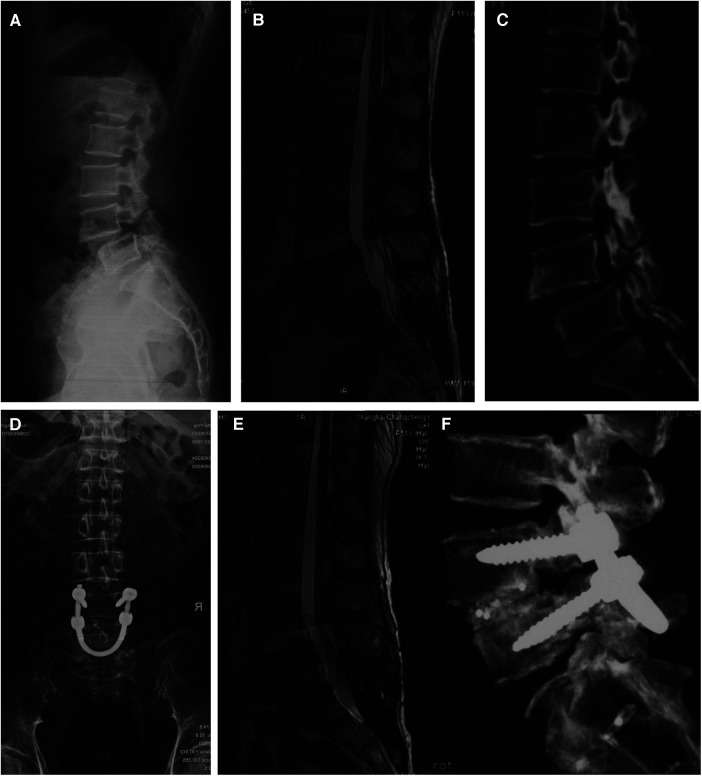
Preoperative and postoperative images obtained in a 51-year-old female diagnosed with double-level lumbar spondylolysis. (A–C) Spondylolysis at L4 and L5, and spondylolisthesis at L4. (D–F) Postoperative radiographs.
